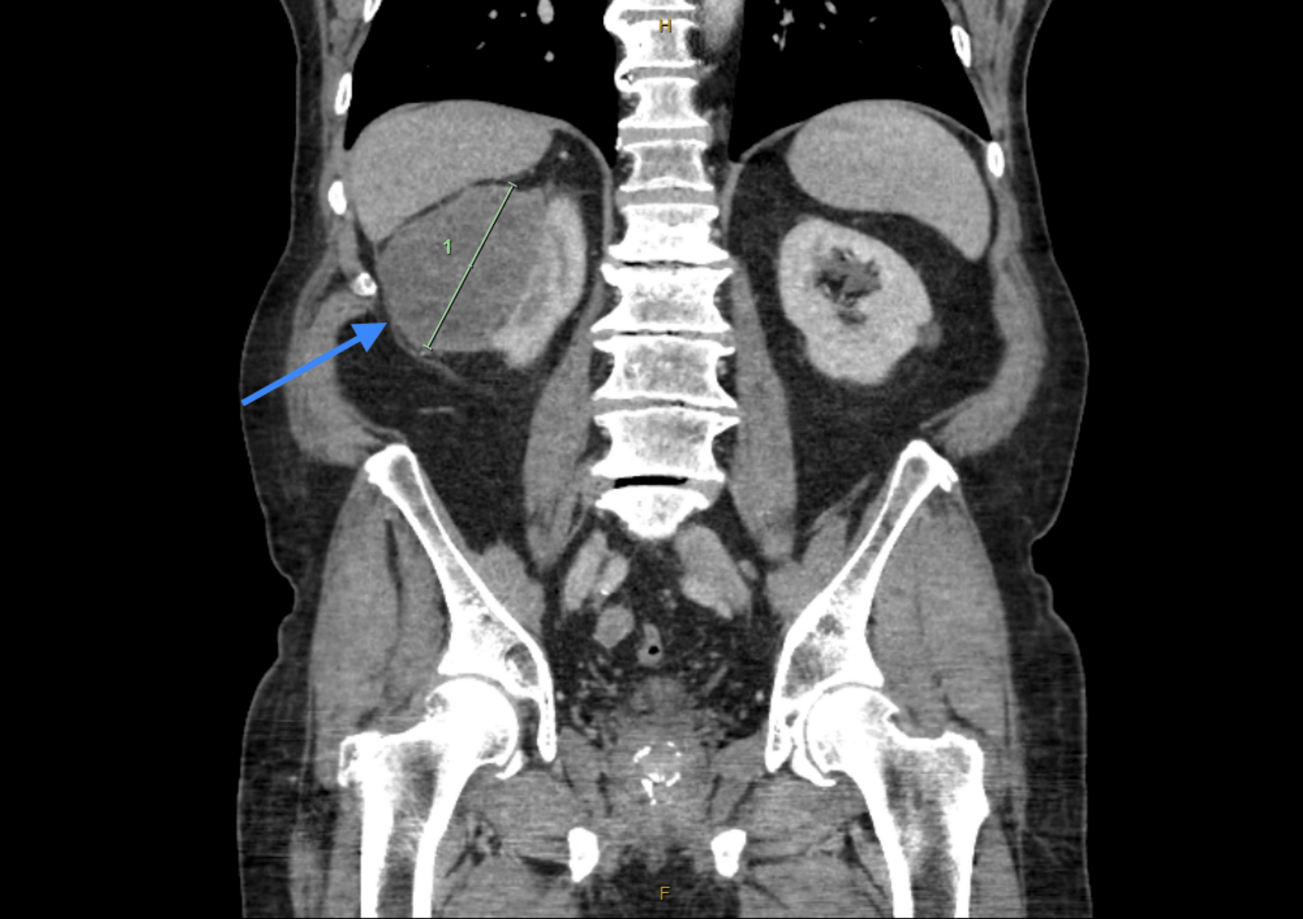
CT abdomen and pelvis with intravenous contrast demonstrating 96 mm mass in the right kidney (arrow).
